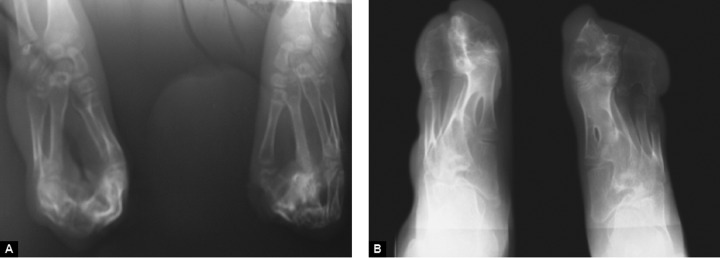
(A) Radiographs of hand and (B) radiographs of feet
